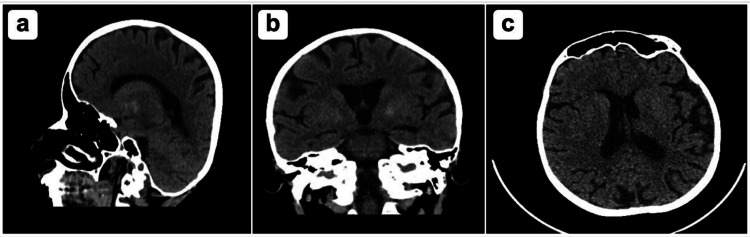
CT brain without contrast showed: A) no acute intracranial hemorrhage or ischemic insult. No gross intracranial masses, mass effect or midline shift. B) Gray and white matter differentiation are preserved. No evolving hydrocephalus. Mild asymmetric hyperdensity in the basal ganglia related to mineralization. C) Hyperpneumatization of left petrous apex. Paranasal sinuses are clear. Partial opacification of the right mastoid air cells. No CT evidence of acute intracranial insult.
